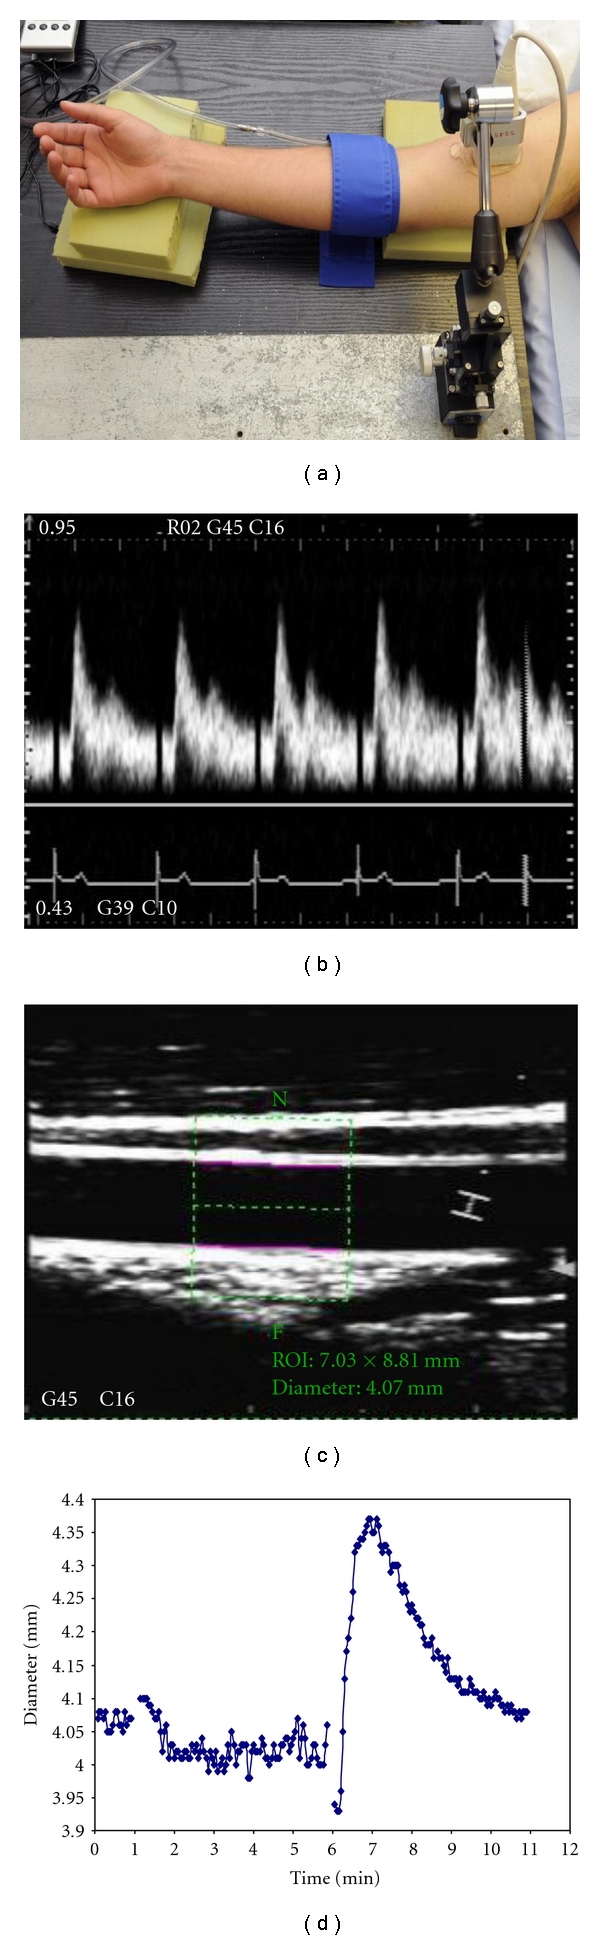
Setup and representative data for ultrasound evaluation of brachial FMD. (a) A sterotatic clamp with micrometer adjustment is used to hold the ultrasound probe. The occluding cuff is positioned just below the elbow. (b) Doppler trace showing flow velocity profile during early reactive hyperemia. (c) B-mode image of brachial artery demonstrating selection of region of interest and edge-detection analysis with Brachial analyzer software package. (d) Brachial artery diameter profile during FMD protocol as measured using brachial analyzer the software package (0-1 minute baseline, 1–6 minutes cuff up, 6–11 minutes response to reactive hyperemia (FMD)).
